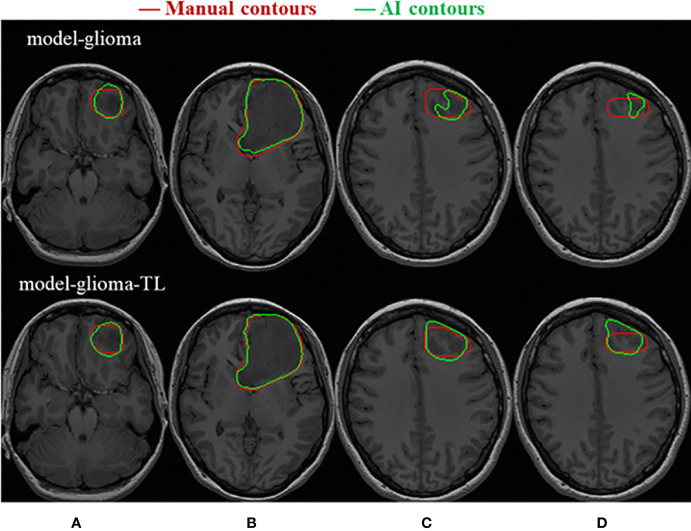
Visualization of the test samples for the two models. The performance of model-glioma-TL was superior to that of model-glioma in the autosegmentation of glioblastoma GTVs, especially in the recognition of the small GTV in the upper and lower MRI slices (A, D) and the boundary delineation of the GTV contours in the intermediate MRI slices (B, C).
